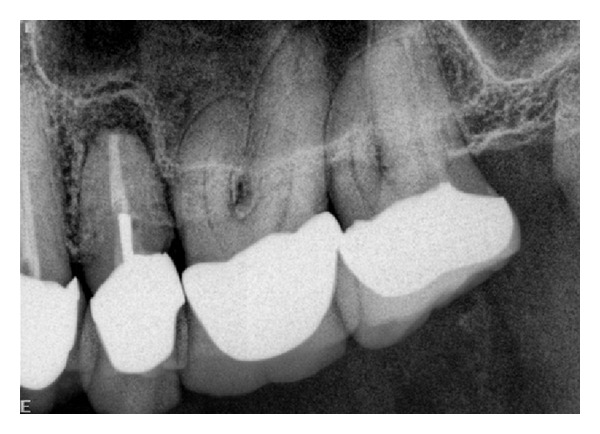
Intraoral periapical radiograph of second premolar tooth used in the study.
